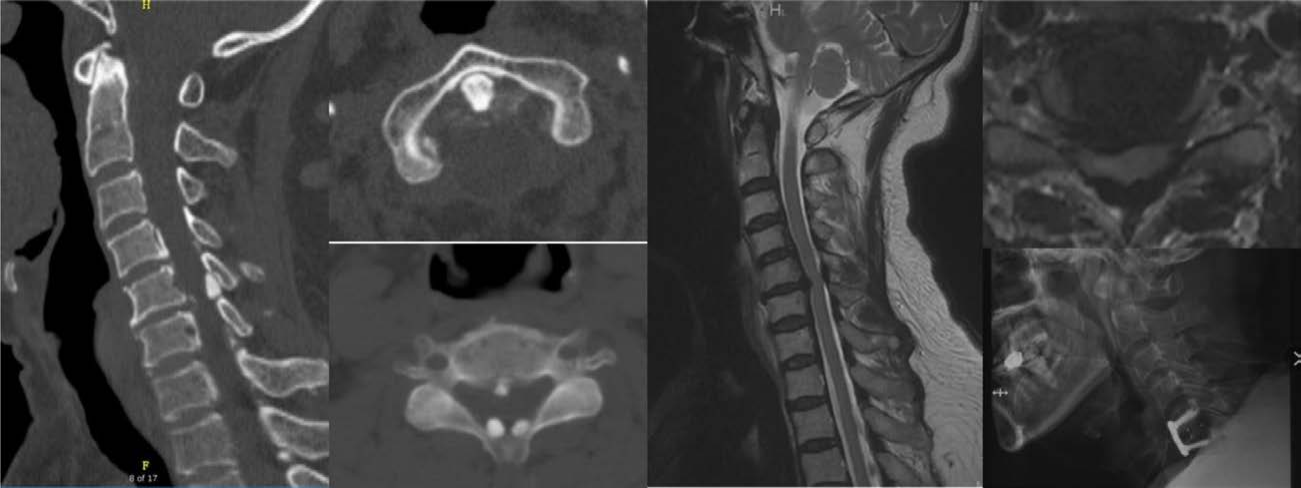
Cervical CT revealed calcification of the transverse ligament of atlas and ligamentum flavum calcification (predominantly at C5-6). Cervical MRI reveals C5/C6 disc herniation with ligamentum flavum hypertrophy causing spinal cord compression. The patient underwent C5/6 anterior cervical discectomy and fusion surgery. CT = computed tomography, MRI = magnetic resonance imaging.
